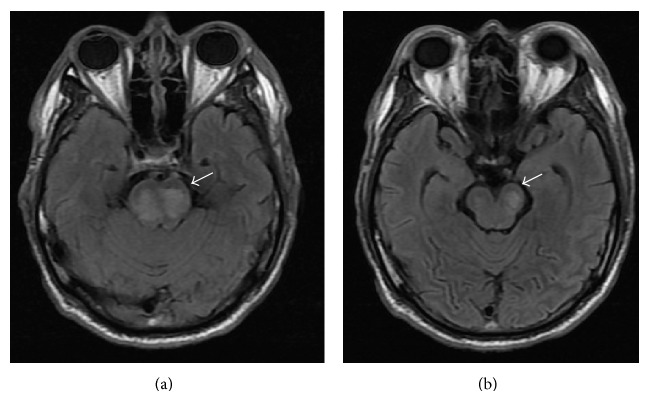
MRI with increased signal intensity on T2-weighted FLAIR images in the medulla oblongata and central pons (arrows).
